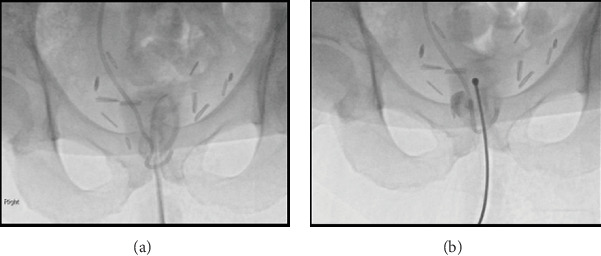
(a, b) Interventional radiology/urology fluoroscopy suite X-ray of the knot and attempt to straighten the knot with introducer following wire attempt.
